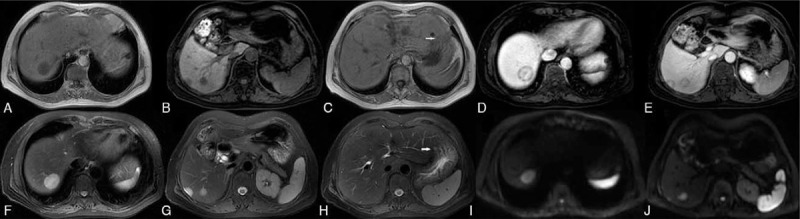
Magnetic resonance imaging (MRI). (A–C) Three hypointense lesions on T1-weighted images (T1-WI); (D,E) central enhancement with peripheral low-signal intensity after the administration of contrast (target-like lesions); (F–H) hyperintense signals on T2-weighted images (T2-WI); (I,J) mild high-signal intensity on diffusion-weighted images (DWI). The “Lollipop sign” (red circles) is a combination of 2 structures: the well-defined tumor and the adjacent occluded vein.
